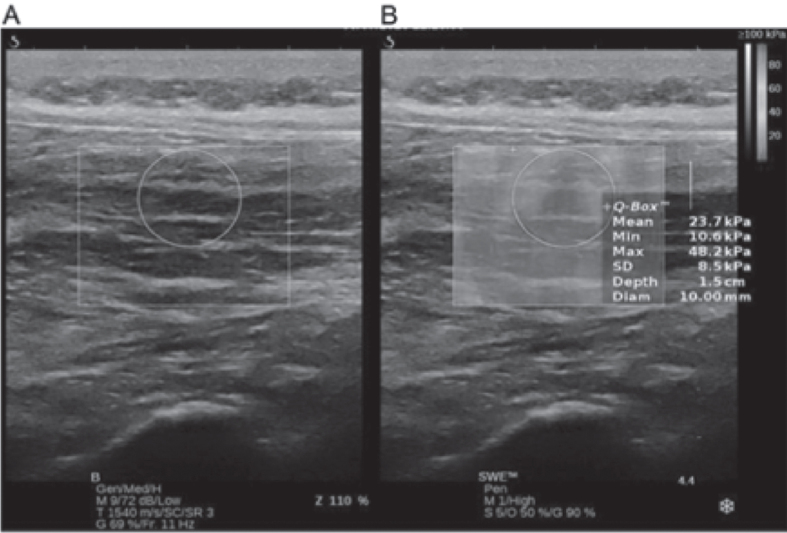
Measurement by shear wave elastography. A: B-Mode ultrasound. B: Overlays shear wave elastography to B-Mode ultrasound and measures Young’s modulus in the Q-Box^™^.
